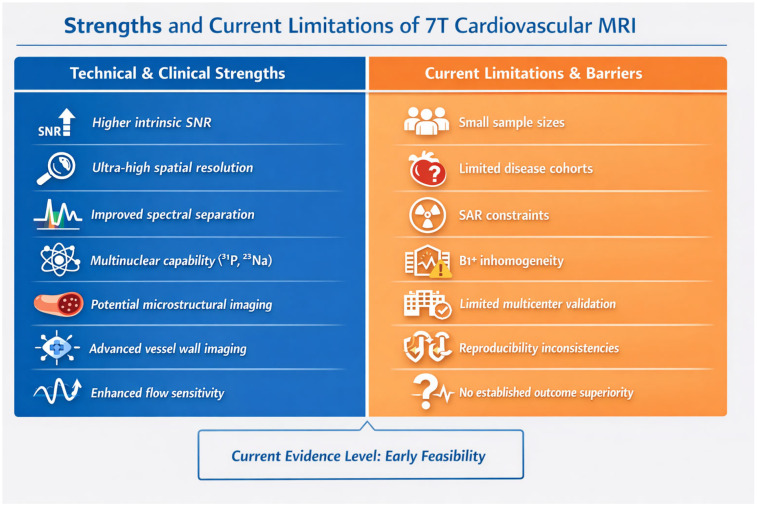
Strengths and current limitations of 7 T cardiovascular MRI.
